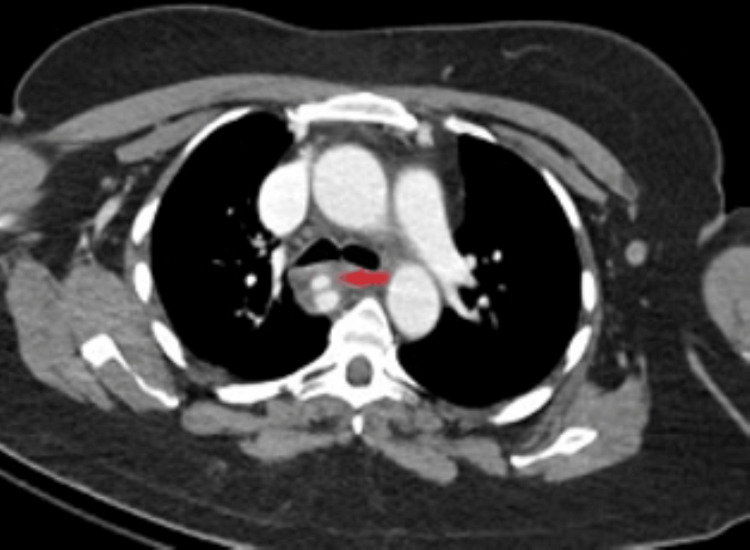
CT with contrast showing an azygos vein injury and pseudoaneurysm. Red arrow points to the area of injury
